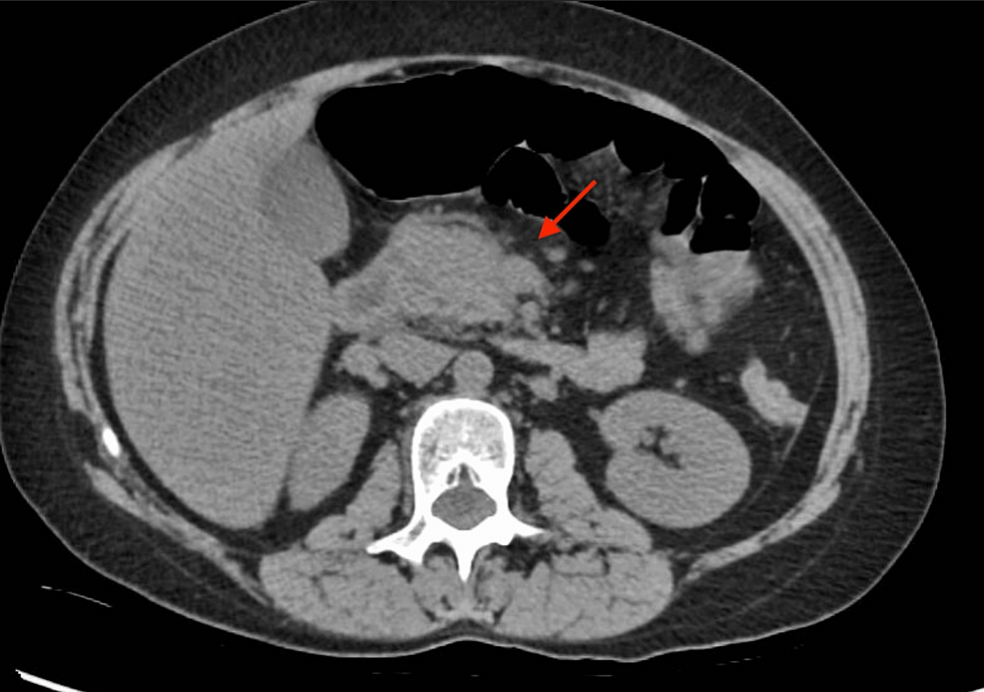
Transverse cross-section CT imaging of the abdomen with the arrow depicting edema surrounding the pancreas.
